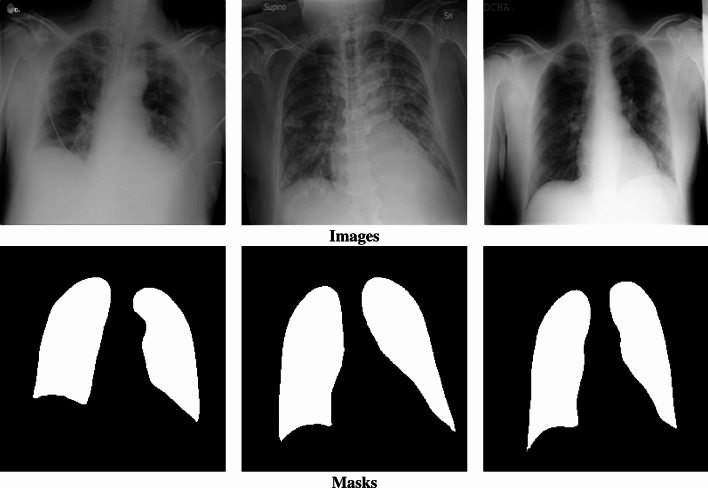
Examples from the COVID-19 Radiography Dataset, including images and their corresponding masks.
